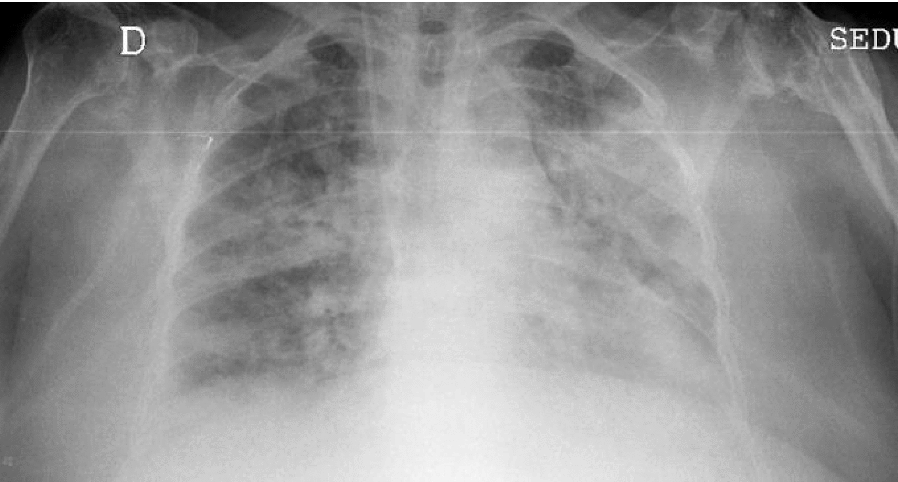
The X-ray shows bilateral and extensive interstitial infiltrates
